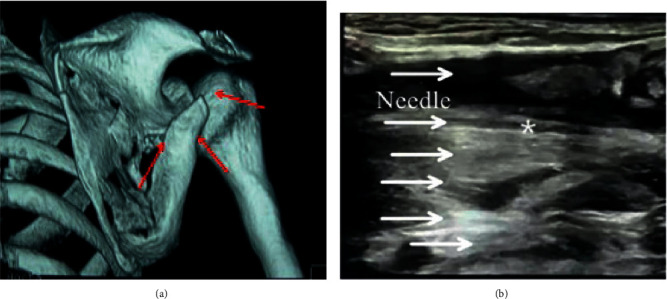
The puncture site (a) of the regional nerve block and the ultrasound anatomy after puncture (b) in the control group. ^*∗*^The bleeding point.
